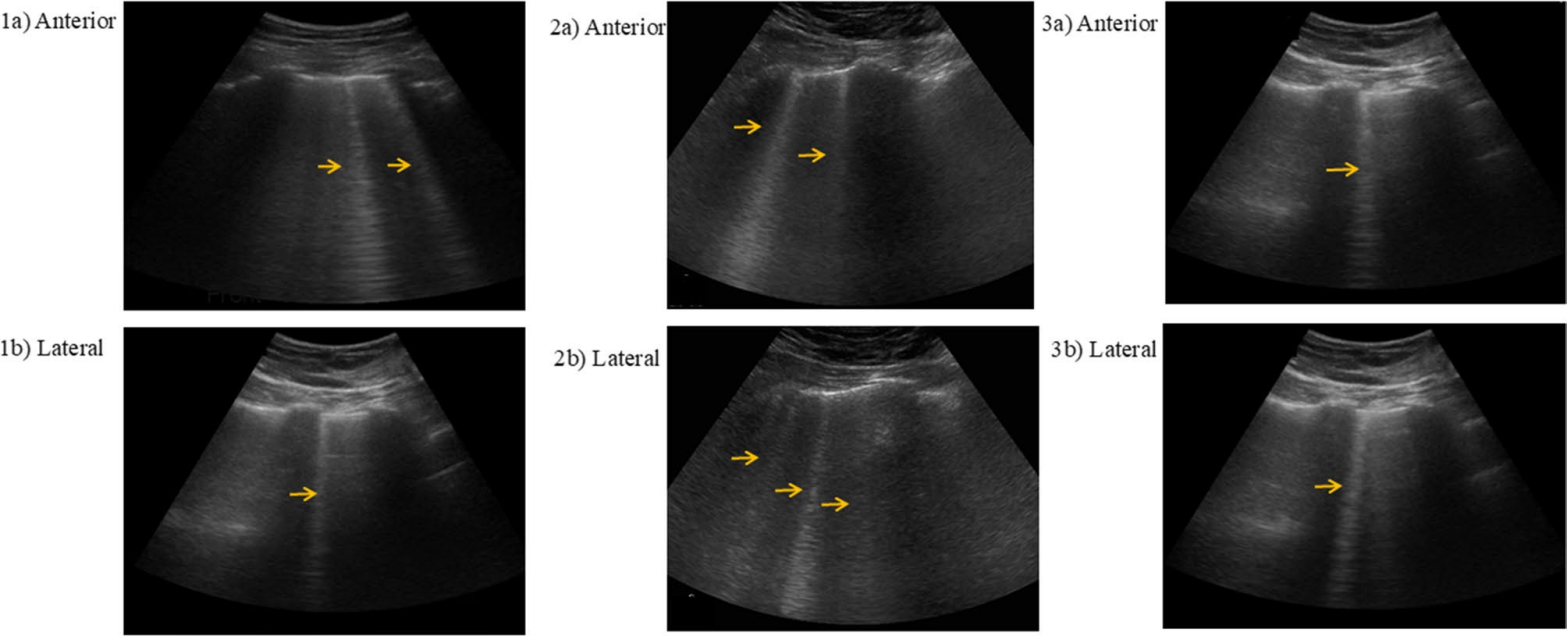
Lung ultrasound at different time after inhaling nitric acid fumes (The arrows refer to B-lines). (1a, 1b) Lung ultrasound 8 h after inhaling nitric acid fumes: Clear laser-like straight lines with “comet tail” characteristics, that is, B-lines, can be seen in the anterior and lateral chest (All three). (2a, 2b) After inhaling for 3 days: The number of B-lines has increased significantly compared with before (All five). (3a, 3b) After inhaling for 5 days: The number of B-lines is less than before (All two)
